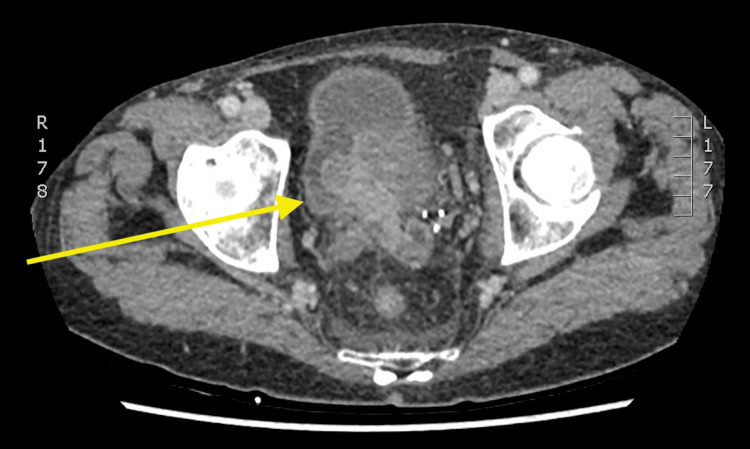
Axial CT images of the patient’s polypous bladder mass and likely primary site of malignancy CT = computed tomography
